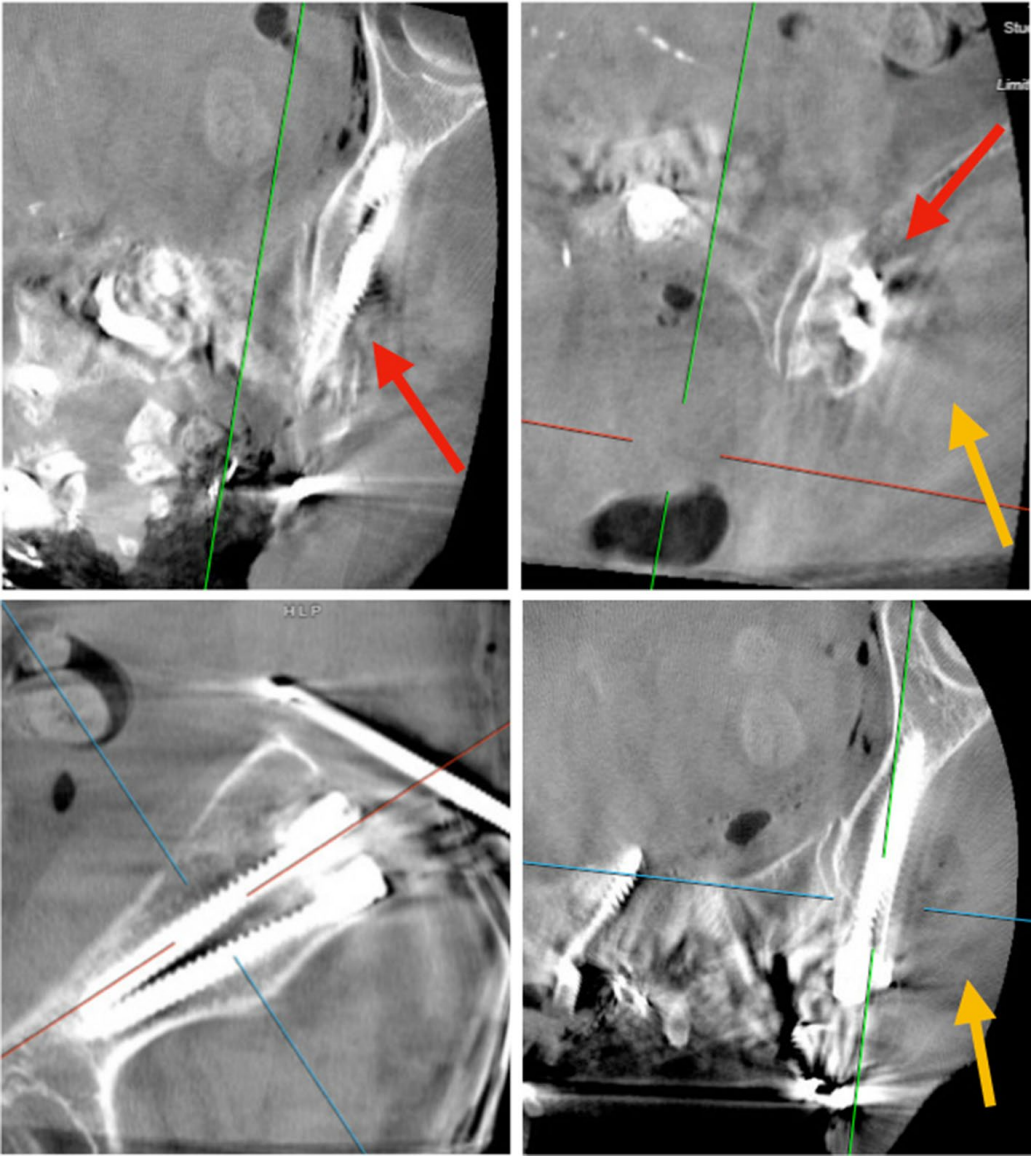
While intra-operative CT scan demonstrated appropriately re-positioned iliac screws, visible were the cortical breach of the lateral wall of the ilium (red arrows) as well as hypointense fluid (gold arrows), likely representing blood, from the originally aberrantly planned trajectory for the proximal iliac screw
